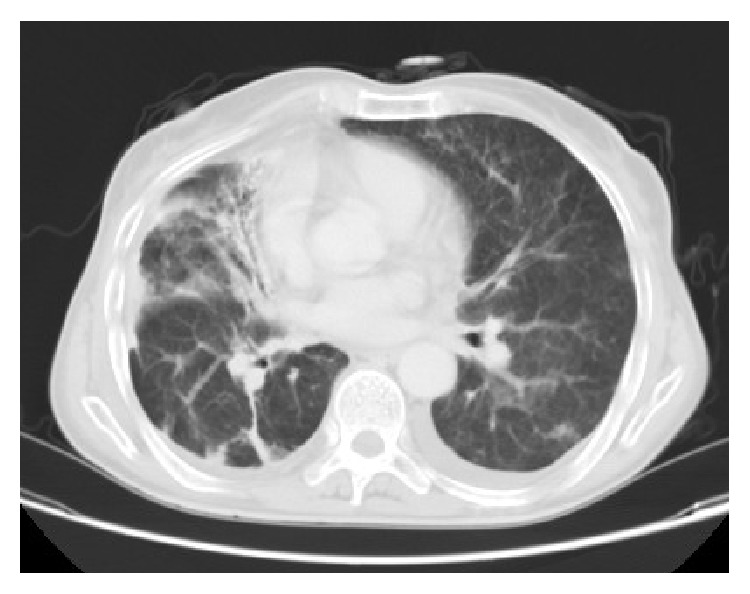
Chest computed tomography: bilateral interstitial thickening and bronchiectasis in the right hemithorax.
